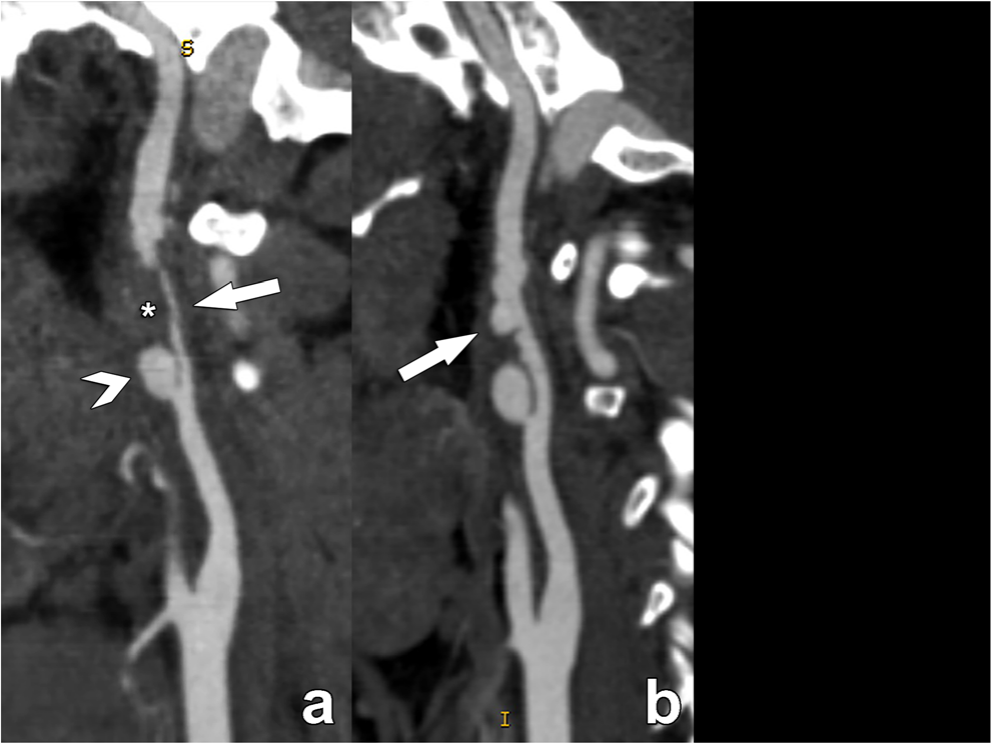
Acute dissection in the supra-bulbar left internal carotid artery (ICA) in a 28-year-old man due to fibromuscular dysplasia, presenting with an acute stroke. The CT angiography examination performed on the day of the acute event reveals a sub-occlusive narrowing of the supra-bulbar left ICA (arrow in a), with a large eccentrically located thrombosed part (asterisk in a), containing a large proximal pseudo-aneurysm (arrowhead in a). Note the complete absence of atherosclerosis in the carotid bulb. On the follow-up examination performed three weeks after the acute event, the thrombus has largely resolved, revealing the typical strings-of-beads sign of fibromuscular dysplasia which was the underlying condition leading to the acute dissection. Fibromuscular dysplasia should always be considered when investigating stroke in young patients
